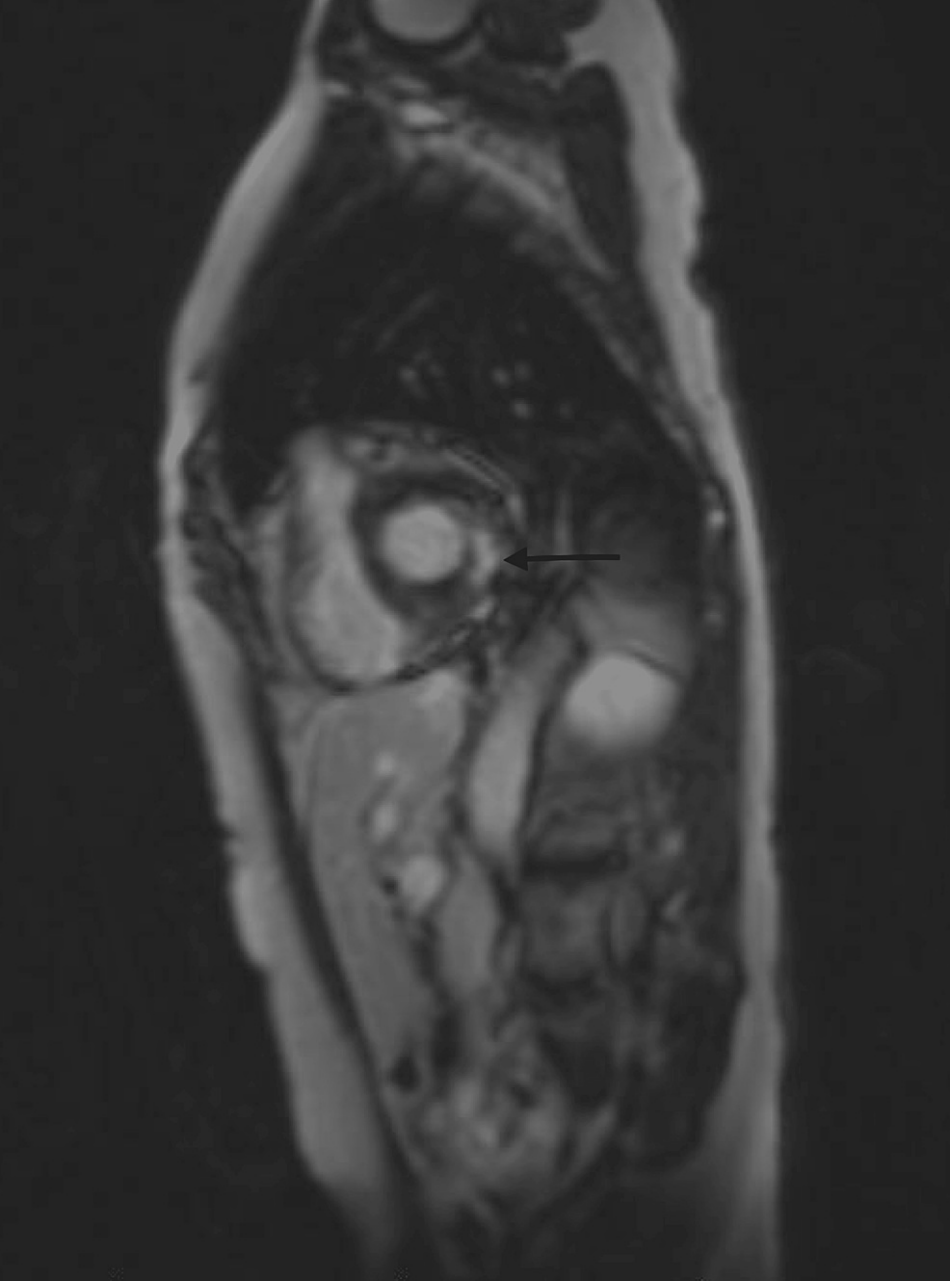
Cardiac magnetic resonance imaging Mid myocardial late gadolinium enhancement in the basal inferolateral wall (arrow) on late gadolinium enhancement images suggestive of myocarditis
